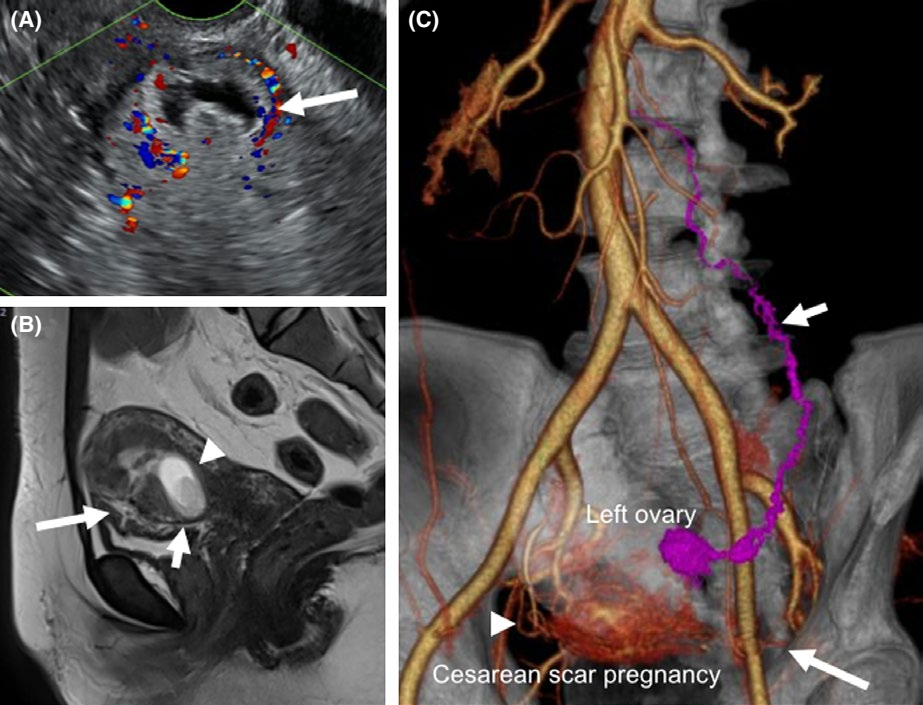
A, Transvaginal ultrasonography showing a heterogeneous mass with perivascular flow in the cesarean section scar (arrow). B, Sagittal T2‐weighted magnetic resonance imaging showing gestational products (arrowhead) implanted in the initial transverse cesarean section scar in the lower uterine segment (short arrow). Vertical scar of the second cesarean section was also noted in the anterior uterine wall (long arrow). C, Three‐dimensional computerized tomographic angiography showing the gestational products receiving aberrant blood supply from the right uterine artery (arrowhead) and anastomosing left ovarian artery (short arrow). In addition, although the main trunk of the left uterine artery was permanently occluded by the platinum coils, some persistent microvascular feeding branches of the left uterine artery (long arrow) were also recognized
